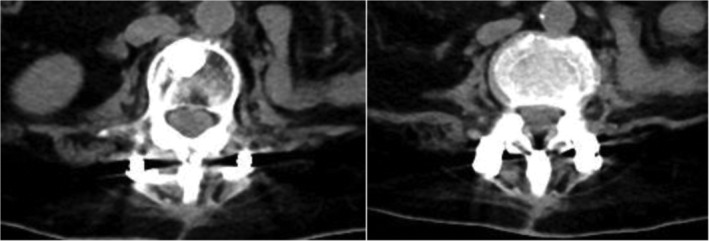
CT Scan after SBRT precision radiotherapy (2.5 months).
